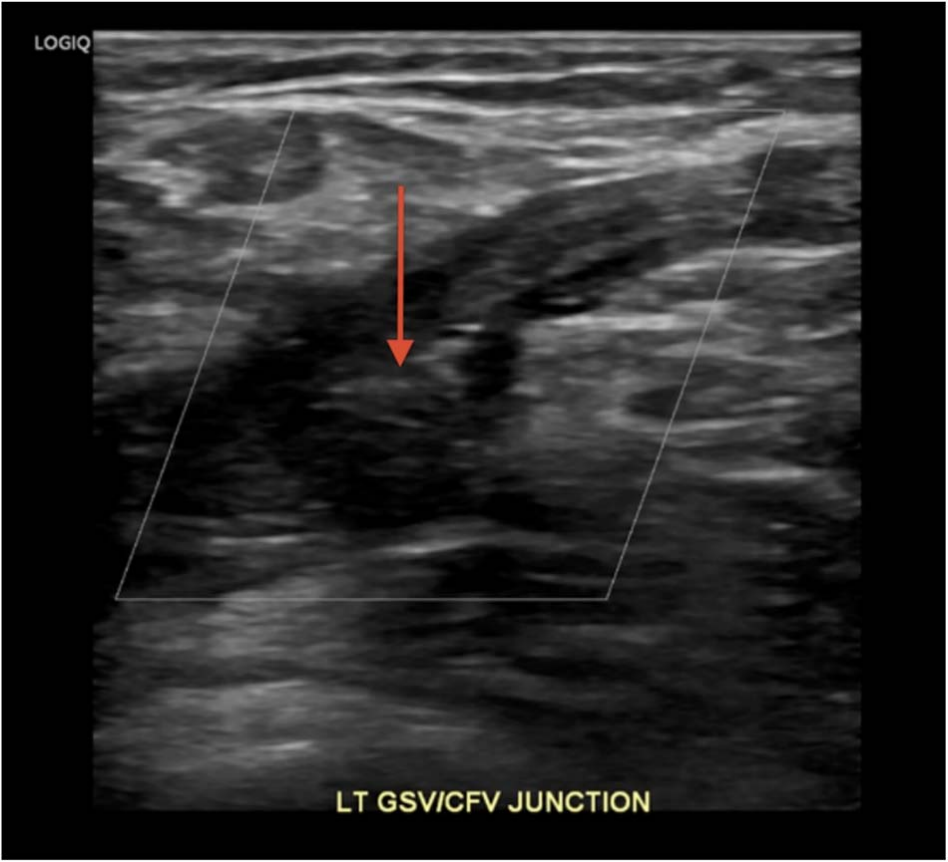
Duplex venous ultrasound of the left lower extremity demonstrating great saphenous vein thrombosis. DVT appears as a hypoechoic area (arrow) indicating turbulent flow in the junction of the great saphenous vein and common femoral vein junction. Abbreviations: LT—Left, GSV—Great saphenous vein, CFV—Common femoral vein.
